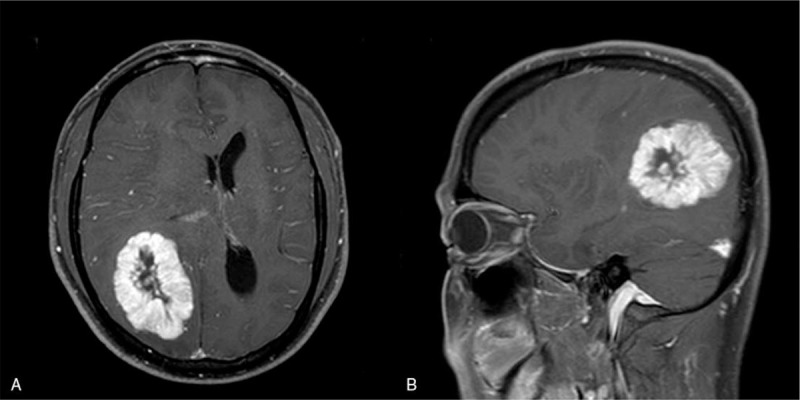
Contrast-enhanced T1-weighted MRI in the axial (A) and coronal (B) planes showed a focal solid tumor in the right parietal lobe (well-defined, round, highly vascular, with surrounding edema, 5.0 × 4.2 cm in size, well-enhanced, with the midline shifted to the left). MRI = magnetic resonance imaging.
